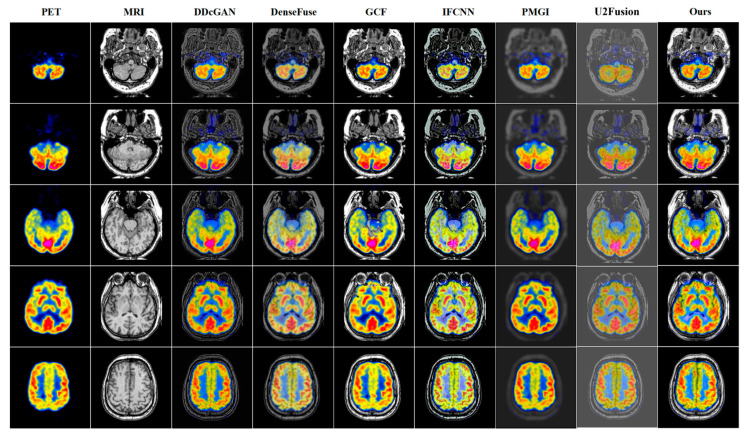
Fusion result of PET-MRI.
